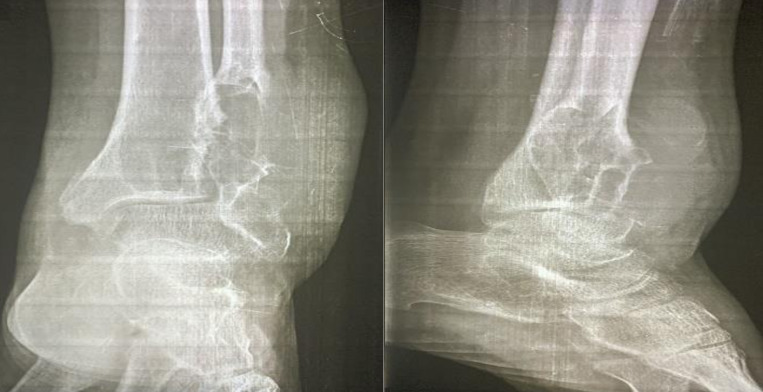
radiographie standard de la cheville gauche objectivant une lésion ostéolytique au niveau de la malléole externe avec rupture corticale partielle et contours irréguliers, envahissant légèrement les parties molles adjacentes
